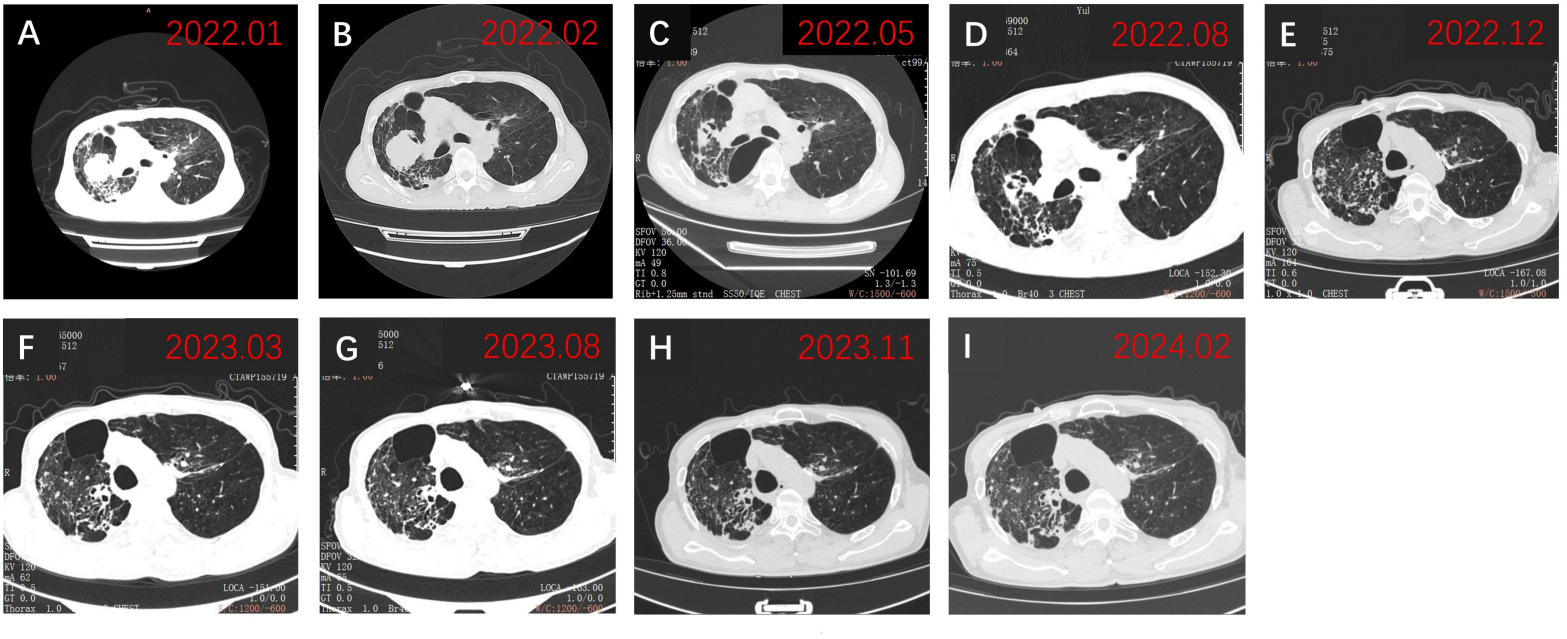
Chest CT findings. (A) Chest CT revealed a tumor on admission in January 2022; (B) Chest CT showed persistent abnormalities before initiation of zimberelimab in February 2022; (C) Chest CT demonstrated a significant decrease in lesion size, achieving PR in May 2022; (D-I) Subsequent chest CTs showed sustained PR of the tumor. PR, partial response.
